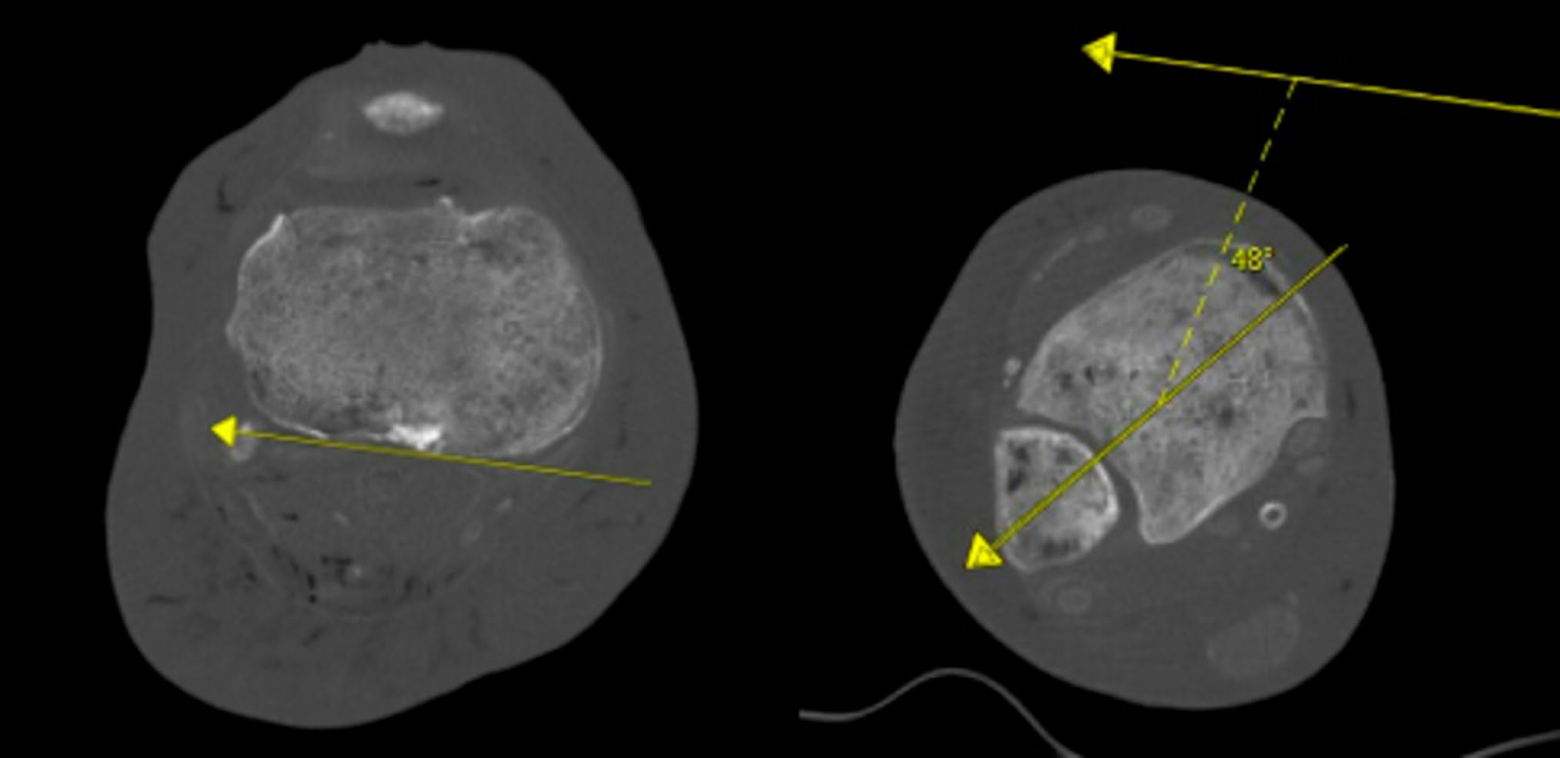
CT assessment of tibial torsion
